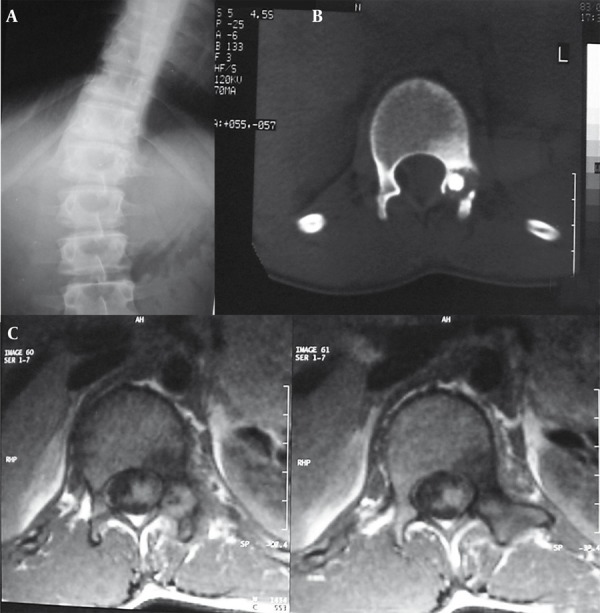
A 22-Year-Old Man With a Complaint of 5-Month Continuous Low Back Pain A, Radiograph revealed a sclerotic region in left pedicle of L1 vertebra along with scoliosis in thoracolumbar region. B, Lumbar CT scan revealed osteolytic and osteoblastic lesion in pedicle of L1 vertebra. C, MRI with T1 weighting demonstrated focal low signal intensity surrounded by soft tissue component replaced pedicle of L1 vertebra on the left side in the axial sections. Diffuse low signal intensity due to edema was seen in the adjacent bone.
